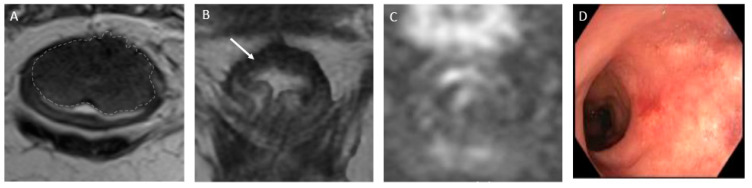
Complete response after neoadjuvant chemotherapy in a 54-year-old man with low rectal adenocarcinoma. (A) Baseline axial T2-weighted MR image shows a low rectal tumor (dotted line). (B) Axial T2-weighted MR image after the completion of neoadjuvant chemoradiotherapy shows a thick hypointense scar at the site of the treated tumor (arrow). No diffusion restriction was present on diffusion-weighted images (C), and no residual malignancy was identified at endoscopy (D). The patient was offered a watch-and-wait (non-operative management) strategy and has been without evidence of disease for 36 months.
